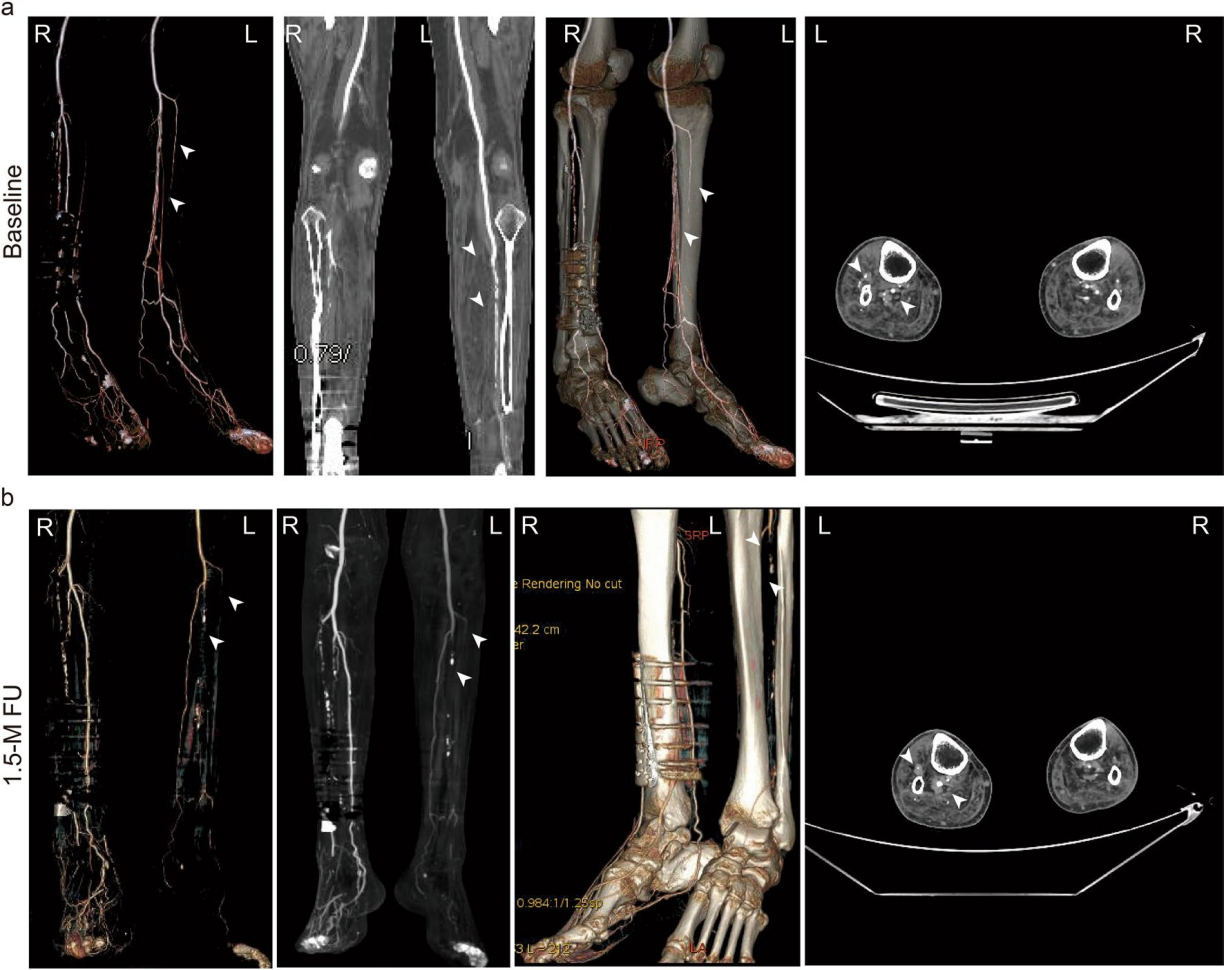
Typical CTA images of the lower limbs of the patients. The severity of angiostenosis of the target lower limbs could not be alleviated after treatments based on the CTA images of the patients. Typical images are shown below for Pt 01 at baseline (a) and at the 1.5-month follow-up (b). Angiostenosis was detected in anterior and posterior tibial arteries as well as arteriae fibularis for Pt 01, especially for anterior tibial artery and arteriae fibularis of his left lower limb (white arrows). No significant improvement was observed in the angiostenosis status of these arteries, in which calcification was detected (white arrows in figures of cross section), at 1.5 months after treatments
